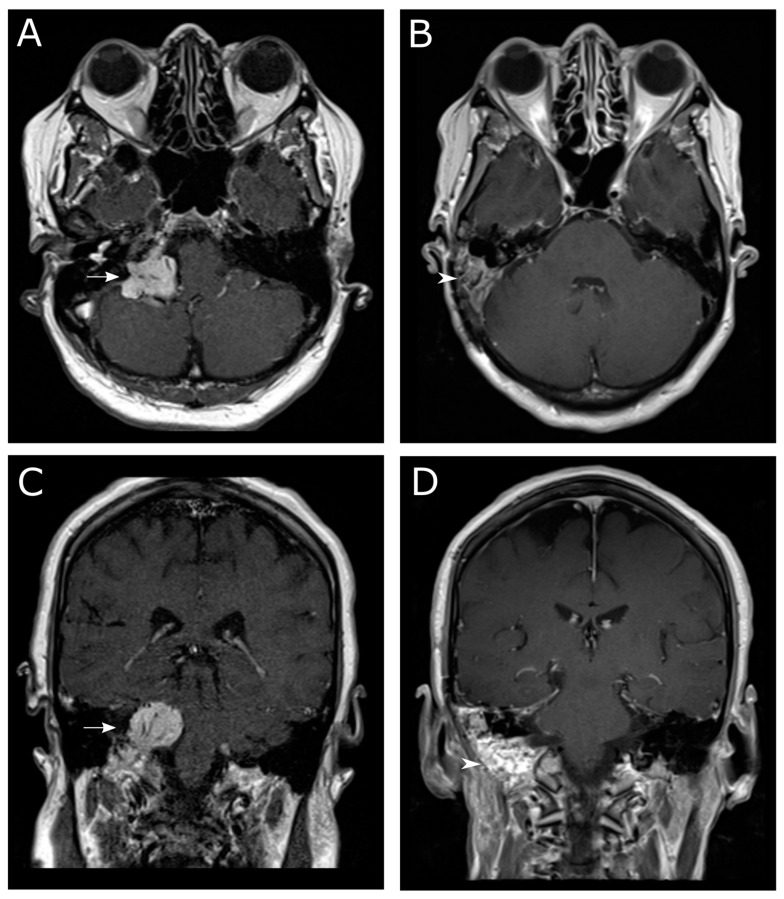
T1-weighted contrast-enhanced MRI of a patient with a Fisch class D jugular paraganglioma who underwent an infratemporal fossa approach for gross total resection of the tumor. The axial pre-surgery image in (A) shows brainstem compression from a contrast-enhancing tumor. Part (B) shows an axial image slightly more cranial compared to the image in (A) showing a fat graft that was used to reconstruct the surgical defect. The coronal pre-surgery image in (C) shows contrast-enhancing tumor extending from the jugular bulb to the cerebellopontine angle. The post-surgery image in (D) shows the removal of intracranial tumor, with a fat graft visible. Arrows in (A,C) indicate tumor. Arrowheads in (B,D) indicate fat graft.
